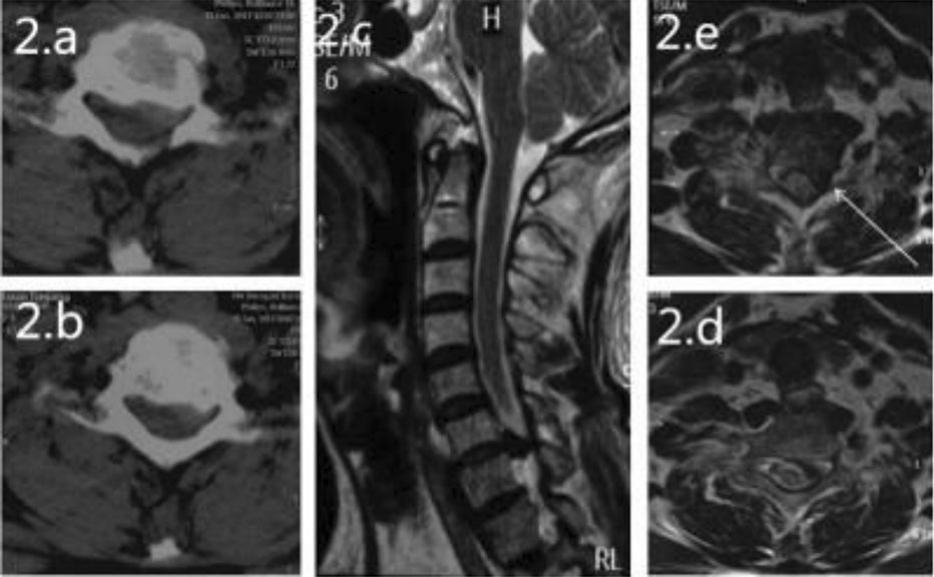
Preoperative MRI and CT. Axial CT (a, b) demonstrates disc herniation pressing on the left nerve root. Sagittal MRI of the cervical spine (c) demonstrates massive disc herniation with nerve root compression at the C6/7 segment. Axial MRI (e, d) showed a herniated disc compressing on the left nerve root (indicated by the white arrow).
